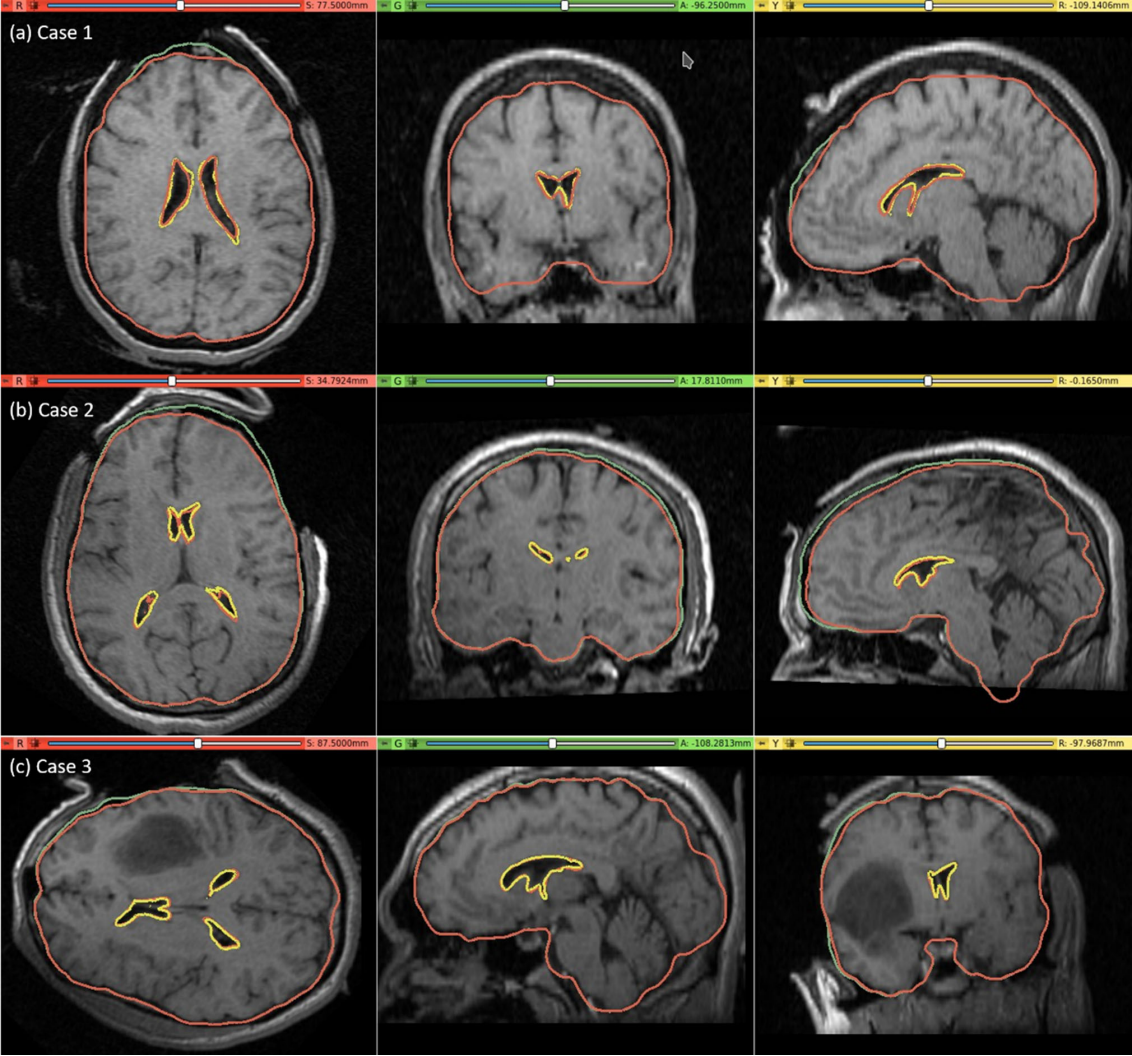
Intra-operative MRI overlaid with contours (red) of the deformed ventricle and brain extracted from the predicted intra-operative MRI which is obtained by warping the pre-operative MRI with the computed transform. Comparison of the brain contours (red) from the predicted intra-operative MRI along with the brain contours (green) extracted from the pre-operative MRI. Predicted red ventricle contours and intra-operative ventricle contours in yellow
